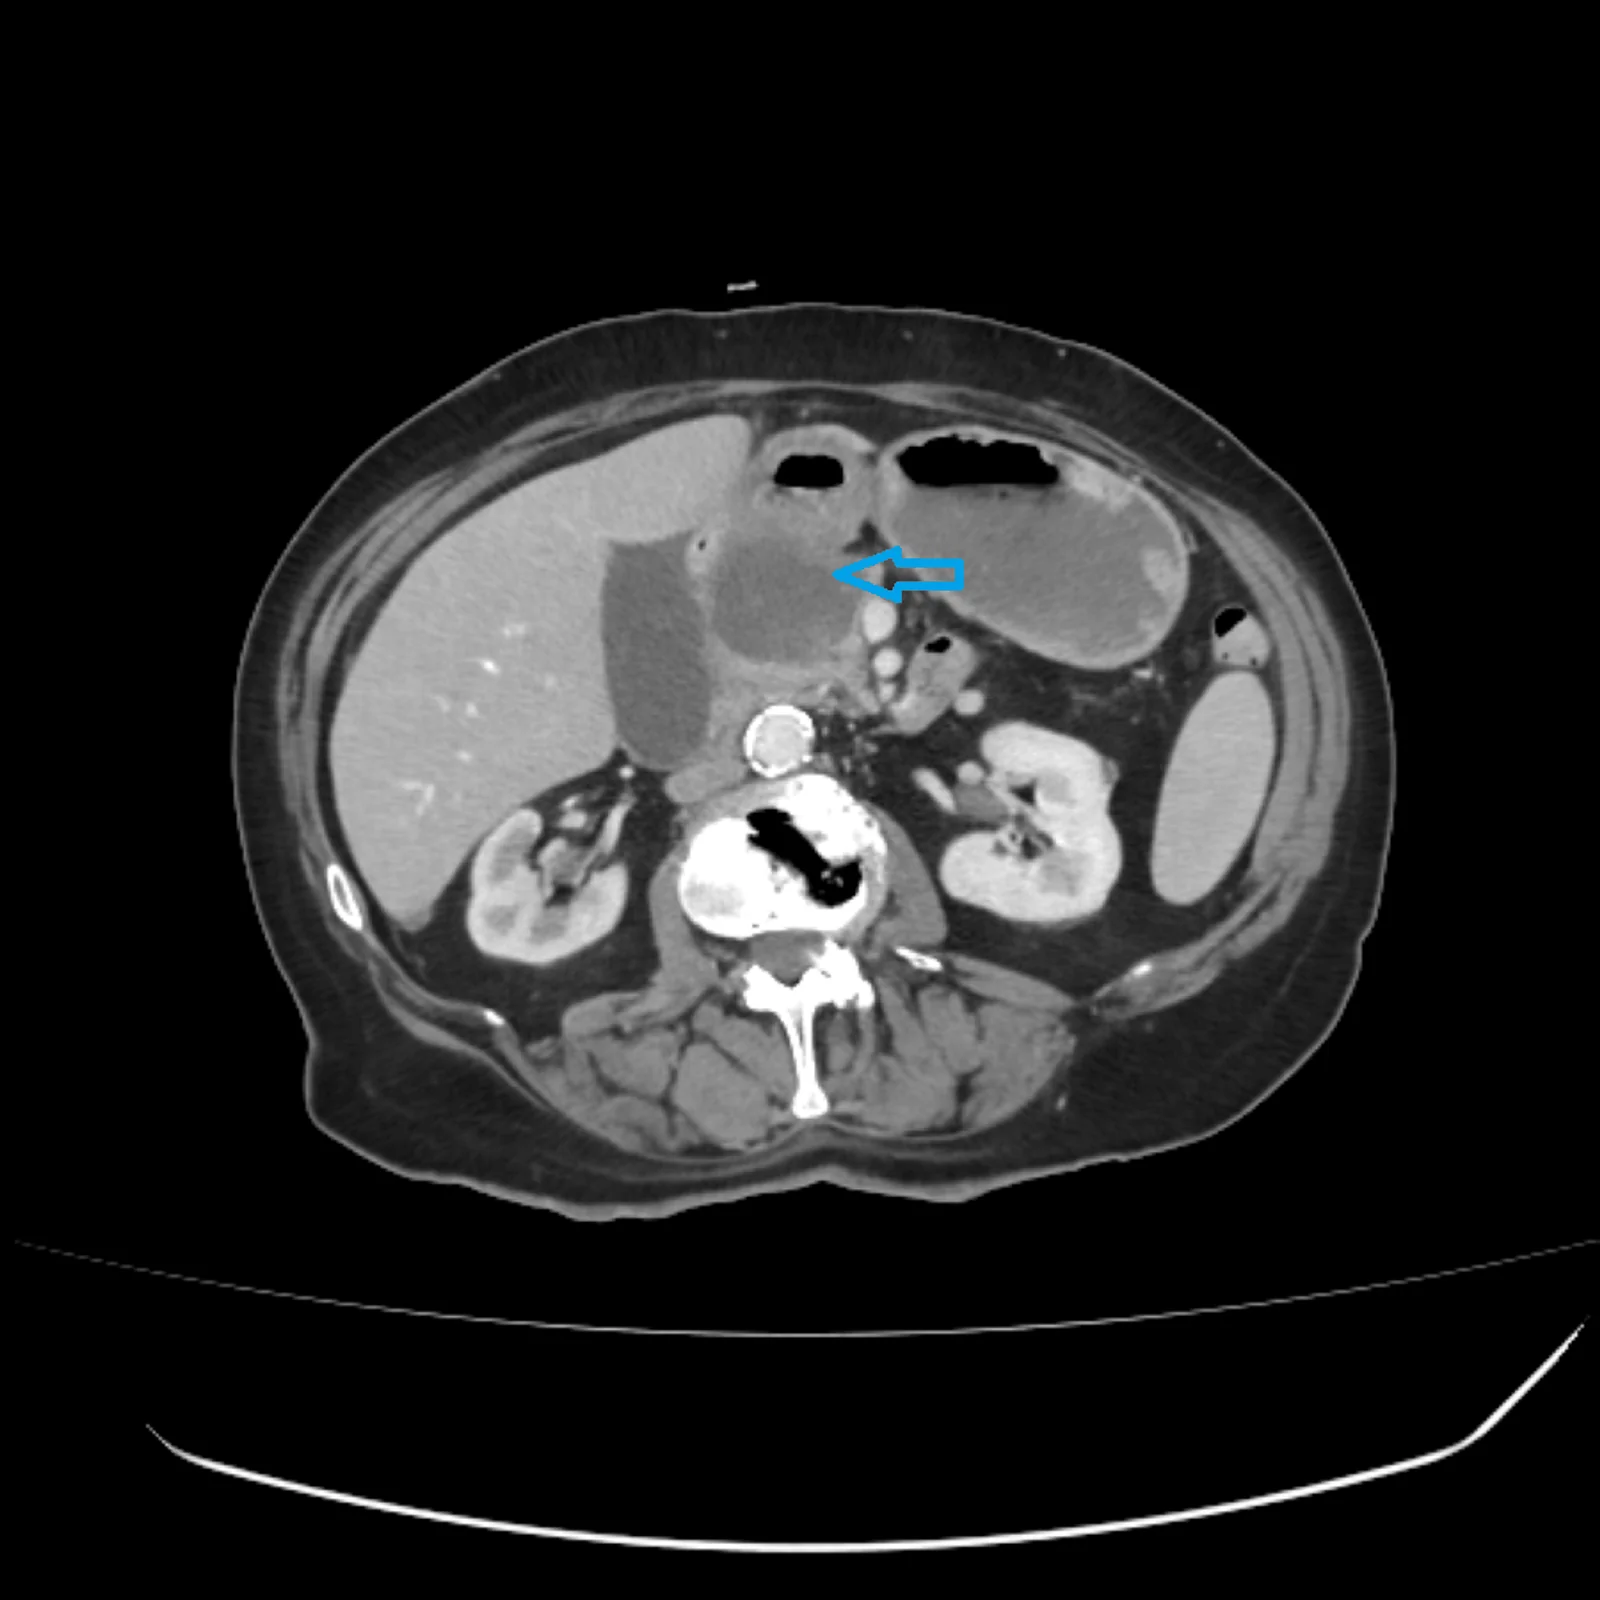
Cross-section of CT abdomen with contrast, with the blue arrow pointing towards large necrotic pancreatic mass.
